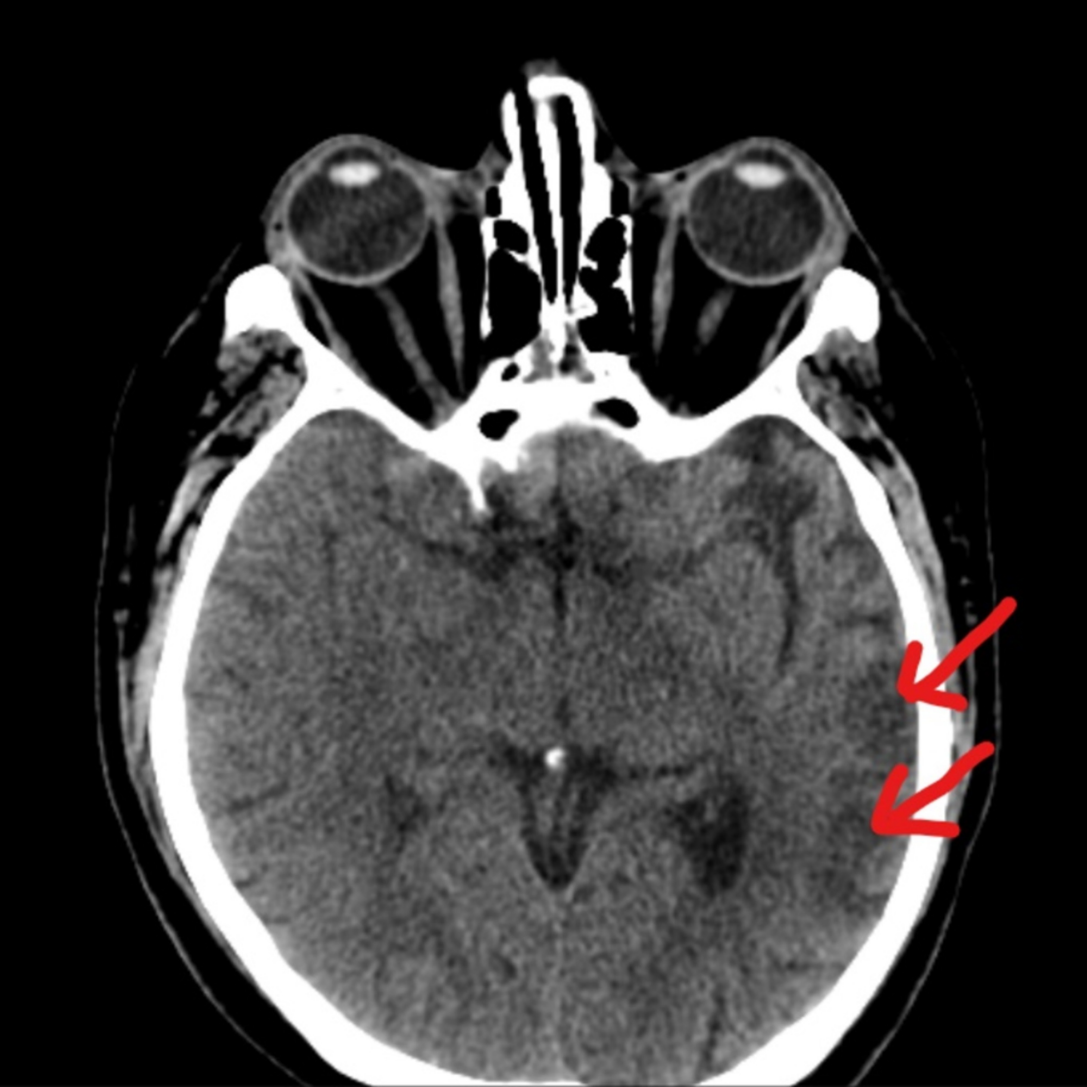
CT of the brain The image shows an acute non-hemorrhagic infarct in the left temporal cortex (arrows) CT: computed tomography
